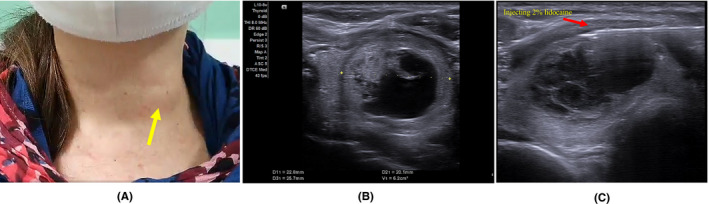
A 48‐year‐old South African woman presented with a left neck mass. (A) A soft mass in the left thyroid lobe, which was moving on swallowing (yellow arrow). (B) A single, mixed echogenicity mass on the left thyroid lobe, with no calcification, no vascularity, and no lymph node involvement. (C) Injecting 10ml of 2% lidocaine at the peri‐thyroidal area under ultrasound guidance (red arrow)
